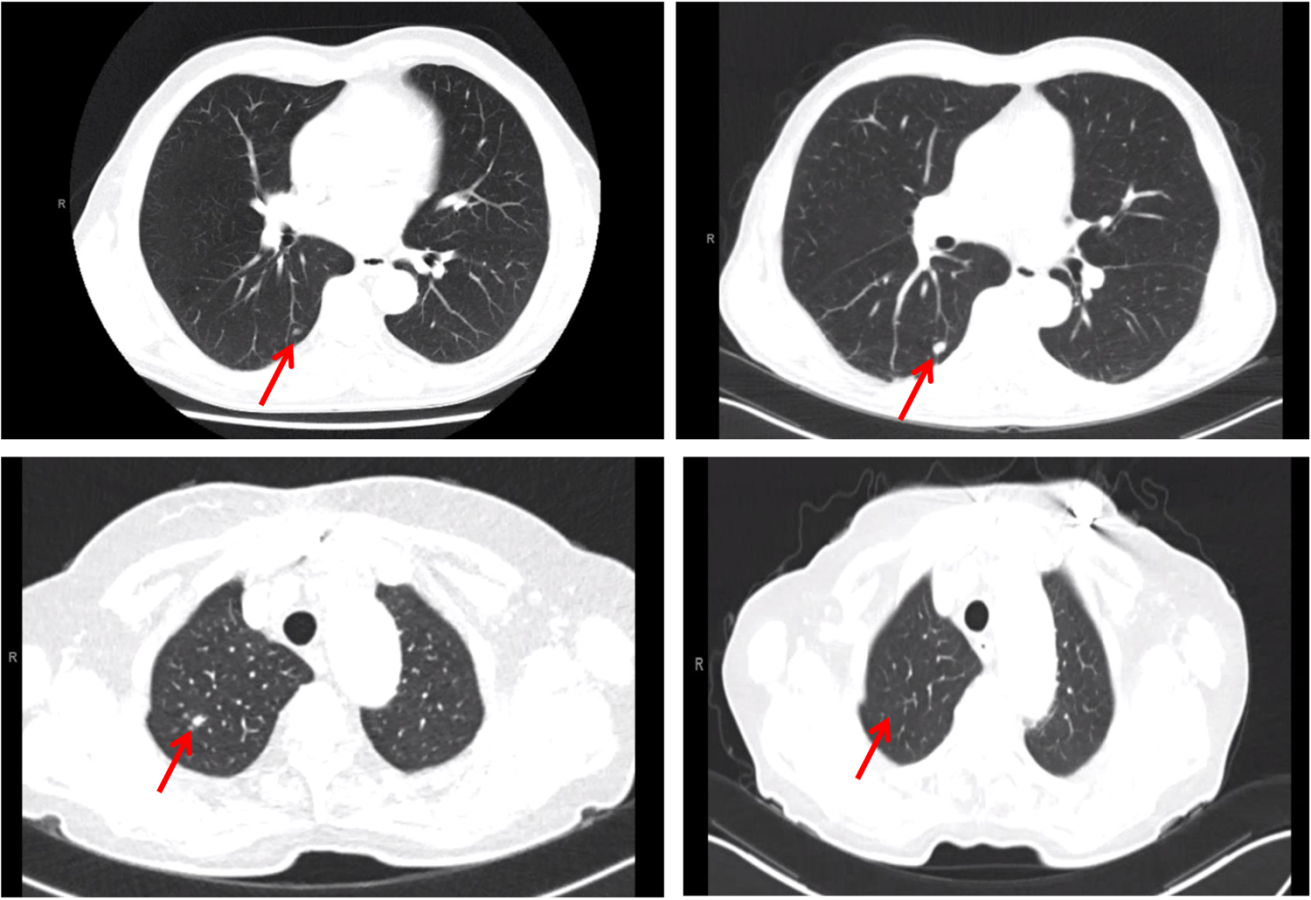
Staging (left) and follow‐up (right) computed tomography scans of patients with nodule too small to characterize (up), and benign nodule (under) [Color figure can be viewed at wileyonlinelibrary.com]
